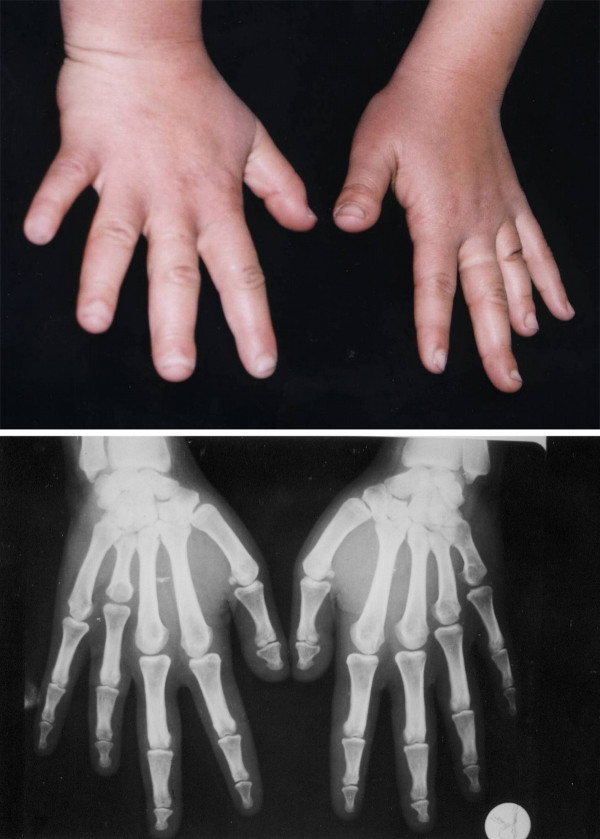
Hands and X-ray hands of an Egyptian patient with BDE. Note the short 4th and 5th metacarpals of hands. (Limb Malformations & Skeletal Dysplasia Clinic, MSU, NRC).
